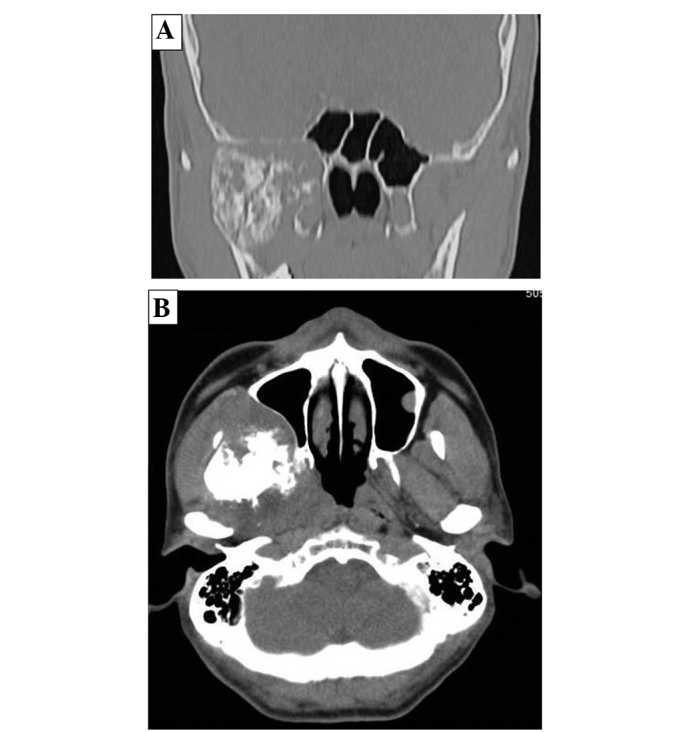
(A) Coronal and (B) axial view of regional computed tomography showing the mass with prominent ossification in the right parapharyngeal space.
